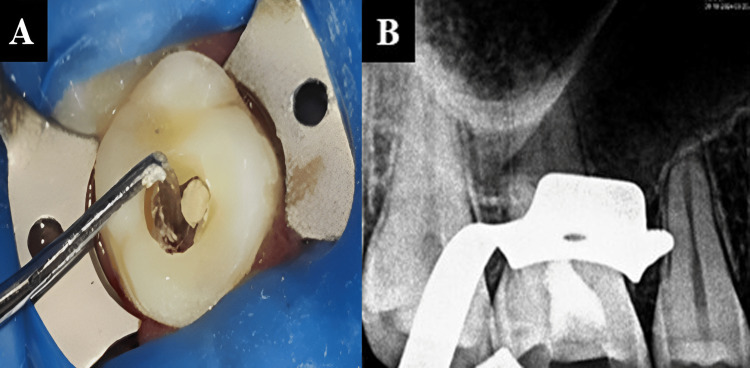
(A) Placement of Bio-C Repair into pulp chamber and (B) radiograph taken after placement of Bio-C repair.
